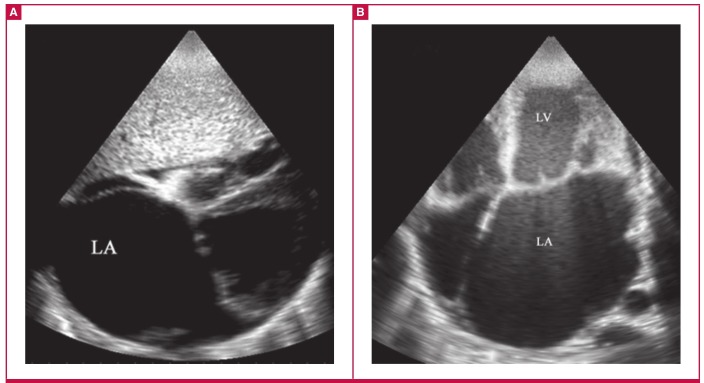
Basic echocardiographic features of advanced left ventricular EMF. Images from a 42-year-old female who presented with intractable heart failure. A is a subcostal view showing the typically huge left atrium occupying half the cardiac size. B is an AP four-chamber view showing apical fibrosis extending to the septum and ventricular walls, leading to severe obliteration of the left ventricular cavity.
